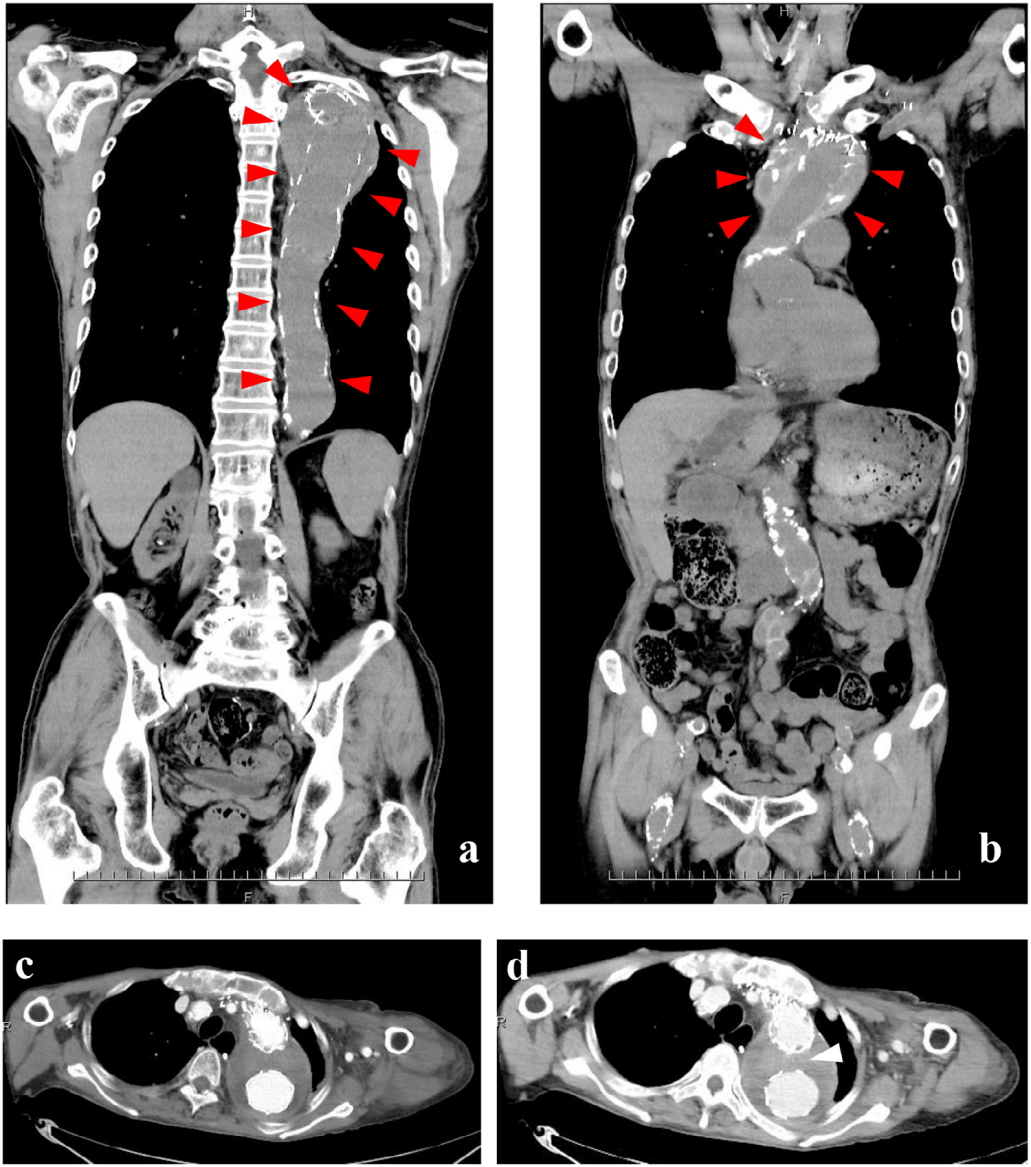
The CT findings of an aortic aneurysm. Abdominal (a) and thoracic (b) aortic aneurysms were replaced with vascular grafts in 2013 and 2019, respectively. The endoleak of aortic aneurysm was revealed by enhanced CT scan on POD 29 in 2023 (c, d, white arrow). CT, computed tomography; POD, postoperative day.
